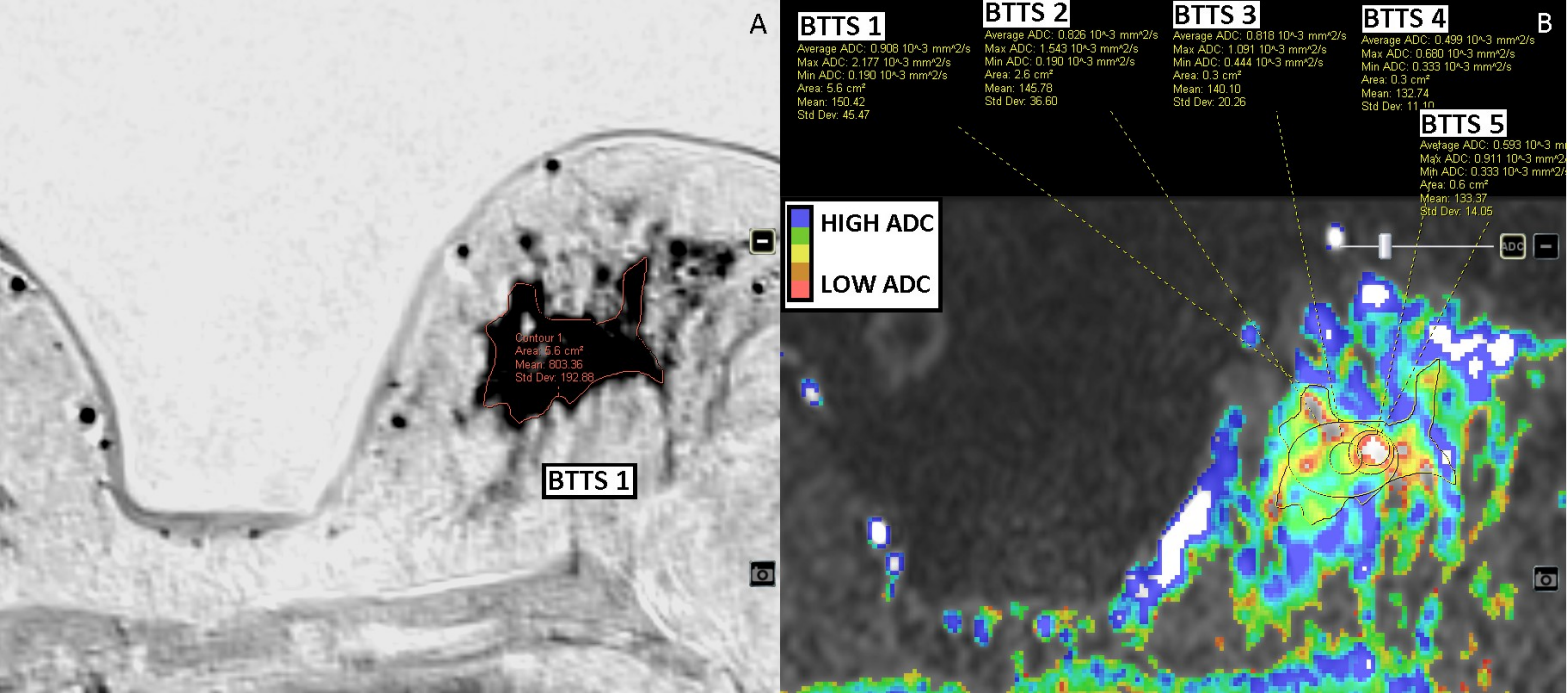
Invasive lobular carcinoma of the left breast, ER positive, PR negative and HER2-neu positive. Surrounding lobular carcinoma in situ. DCE-T1 subtracted images (inverted) (A) and DWI b0-1000 images (B).
